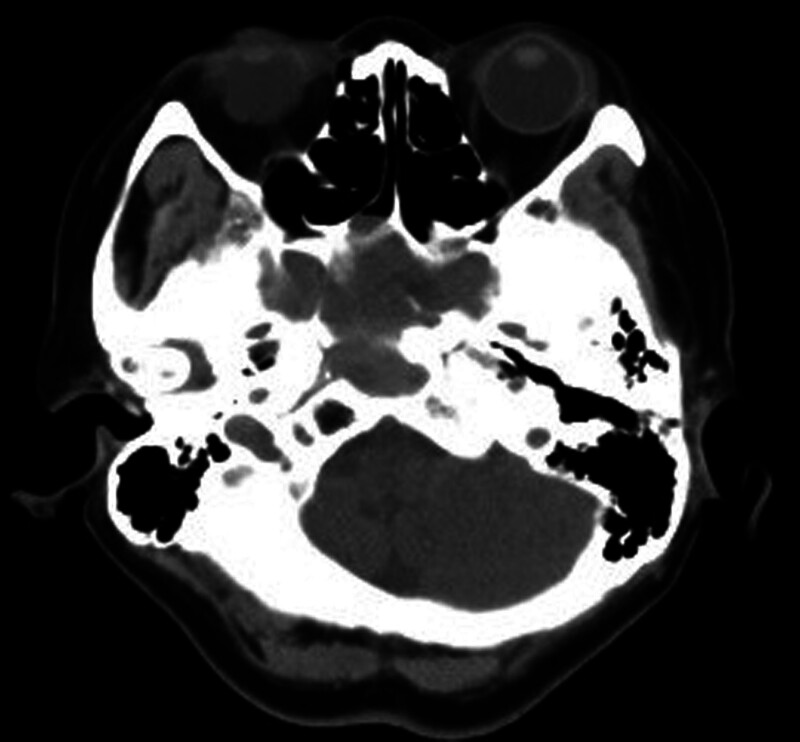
Patient, 70 years old, female, preoperative sinus CT reconstruction horizontal. CT = computed tomography.
